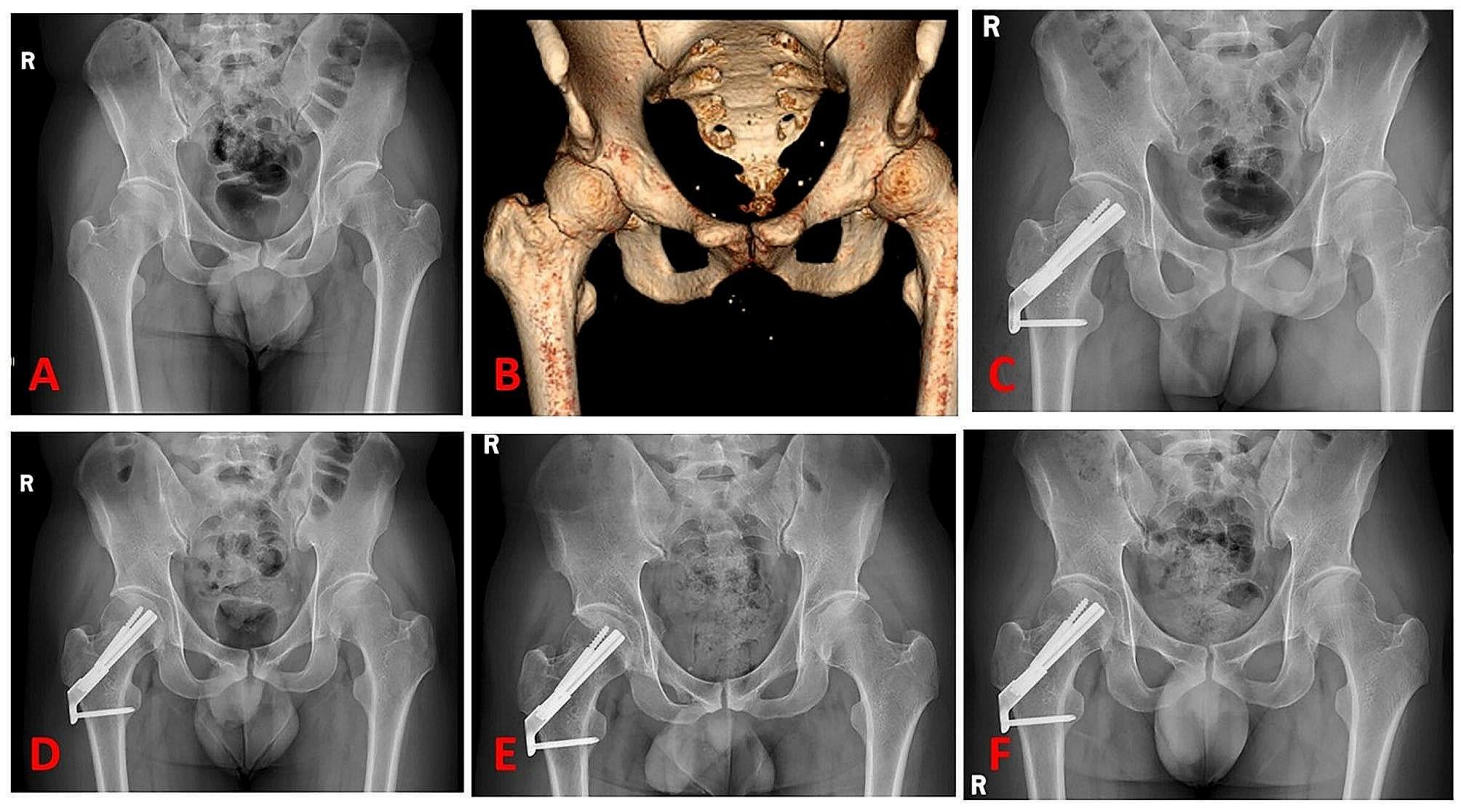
A 45-year-old male patient suffered right femoral neck fracture. (A) and (B) showed the femoral neck fracture. (C), x-ray postoperative. (D), X-ray three months after operation. (E), six months after operation. (F), one year after operation
